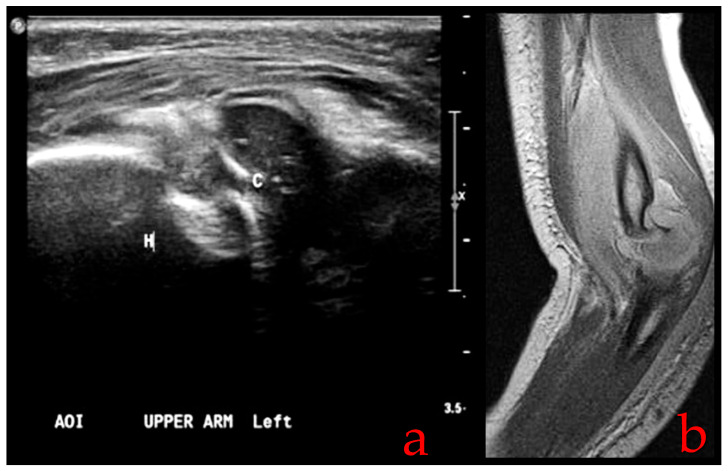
US (a) and MRI (b) images showing transphyseal distal humerus separation.
